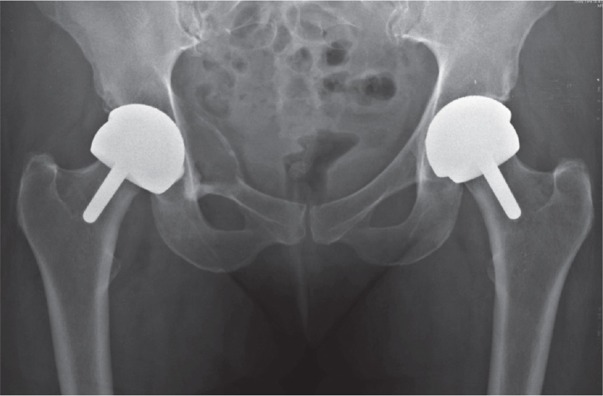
Preoperative radiograph of patient 34 demonstrating extensive osteolysis adjacent to the right acetabular component.
